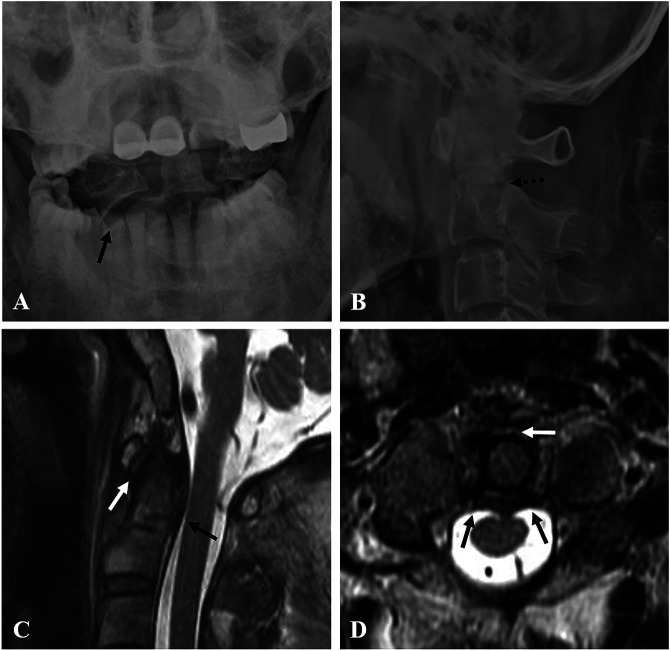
Initial open mouth view (A) and lateral radiograph (B) showing a type 3 dens fracture with angulation (dotted dark arrow) and C1-2 lateral subluxation (dark arrow). Sagittal (C) MRI showing a type 3 dens fracture with intact transverse atlantal ligament (dark arrow) and normal atlanto-dental interval (ADI) (white arrow). Axial (D) MRI showing an intact transverse atlantal ligament (dark arrows) and normal ADI (white arrow).
